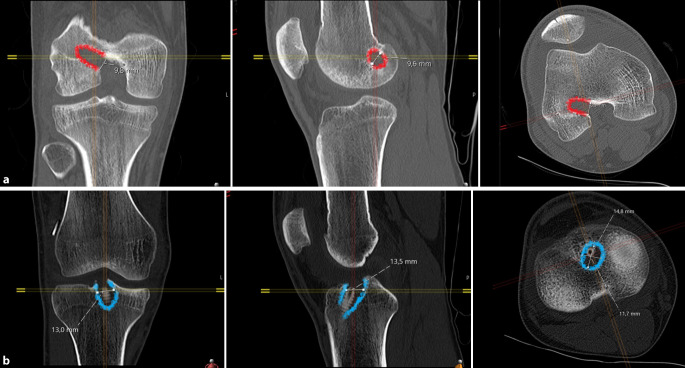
Eine CT-ArthrographieCT-Arthrographie (Arthro-CT) kann zur Diagnostik herangezogen werden, wenn eine MRT-Untersuchung aus medizinischen Gründen (z. B. kardiologische Implantate) nicht möglich ist. Im Gegensatz zur MRT bestehen hier die Notwendigkeit einer intraartikulären Kontrastmittelapplikation und zusätzlich die CT-bedingte Strahlenbelastung. Bei ähnlicher Spezifität ist die Sensitivität jedoch im Vergleich zur MRT geringer. Somit ist die CT-Arthrographie kein Mittel der ersten Wahl zur primären Diagnostik.
